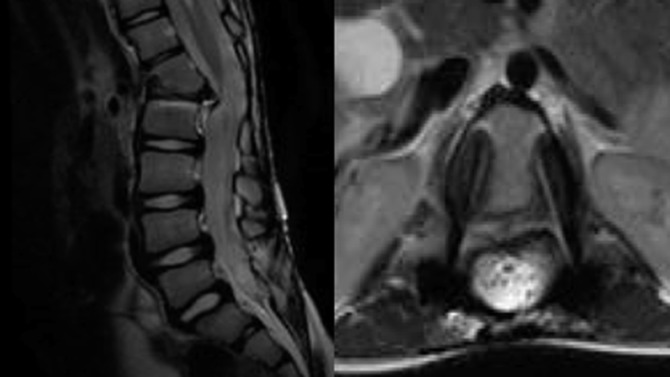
MRI at last follow-up (5 years postoperatively) demonstrating adequate canal space. (Reproduced with permission from the Children's Orthopaedic Center, Los Angeles.)
